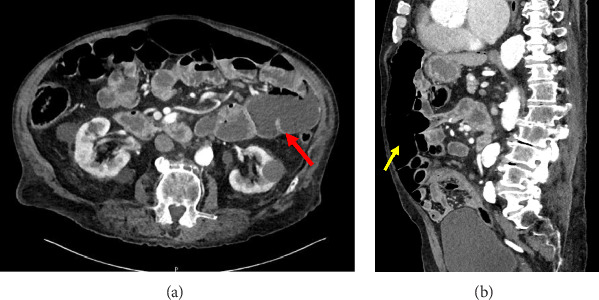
Repeat CT abdomen and pelvis at 8-month follow-up visit: (A) Axial view demonstrating improved pneumoperitoneum and no signs pneumatosis intestinalis, therefore, indicating resolution (red arrow). (B) Sagittal view demonstrating improved pneumoperitoneum (yellow arrow) compared to previous CT scans.
